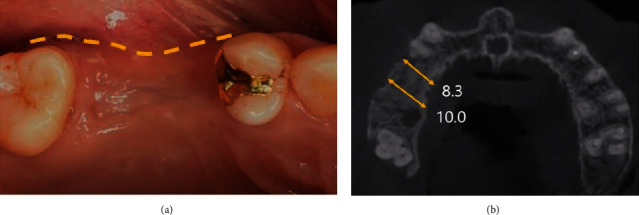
Periodic follow-up at 4 months after operation (Case 1). (a) Intra-oral view. (b) CBCT view. The width of the alveolar bone had increased.
